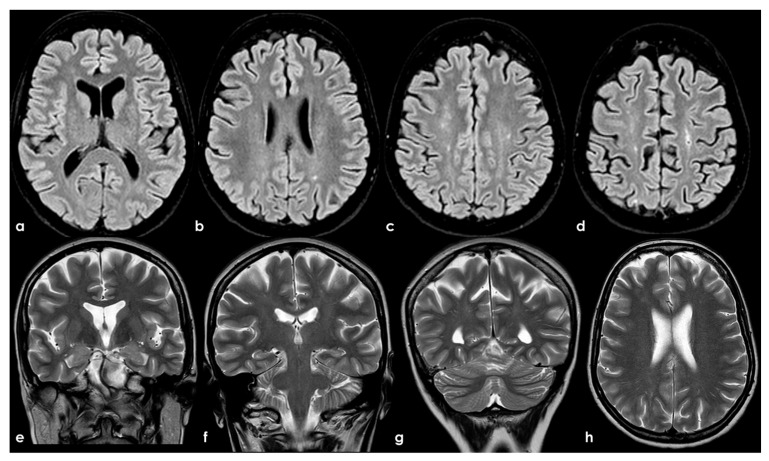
Brain MRI (axial FLAIR in panels (a–d), coronal T2W in panels (a–g), and axial T2W in panel (h)) showing small punctate white-matter hyperintensities in the centrum semiovale, with a trend to watershed distribution (panel (d)), and a mild increase in enlarged perivascular spaces in the subcortical white matter (panels (e–h)).
